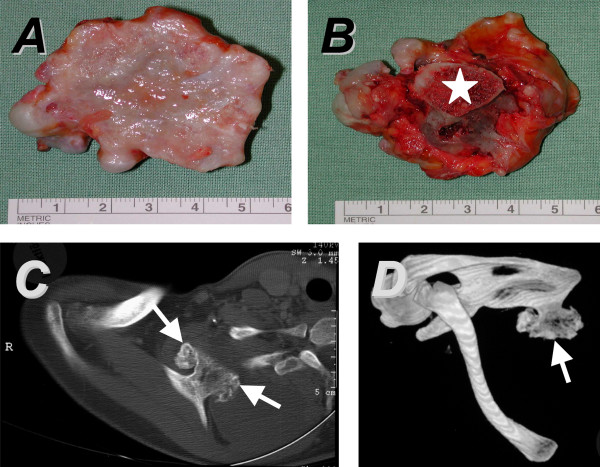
Case 1. The ventral surface of the osteochondroma (A) is covered by the cartilaginous cap. The macroscopic aspect of the dorsal surface of the tumor (B) reveals the bony stalk (star). In the computed tomography (CT) scan, the lesion can easily be identified on the ventral aspect of the right scapula (C; arrowheads). Its localization on the margo superior close to the angulus superior is demonstrated by a three-dimensional reconstruction of the CT images (D; arrow).
